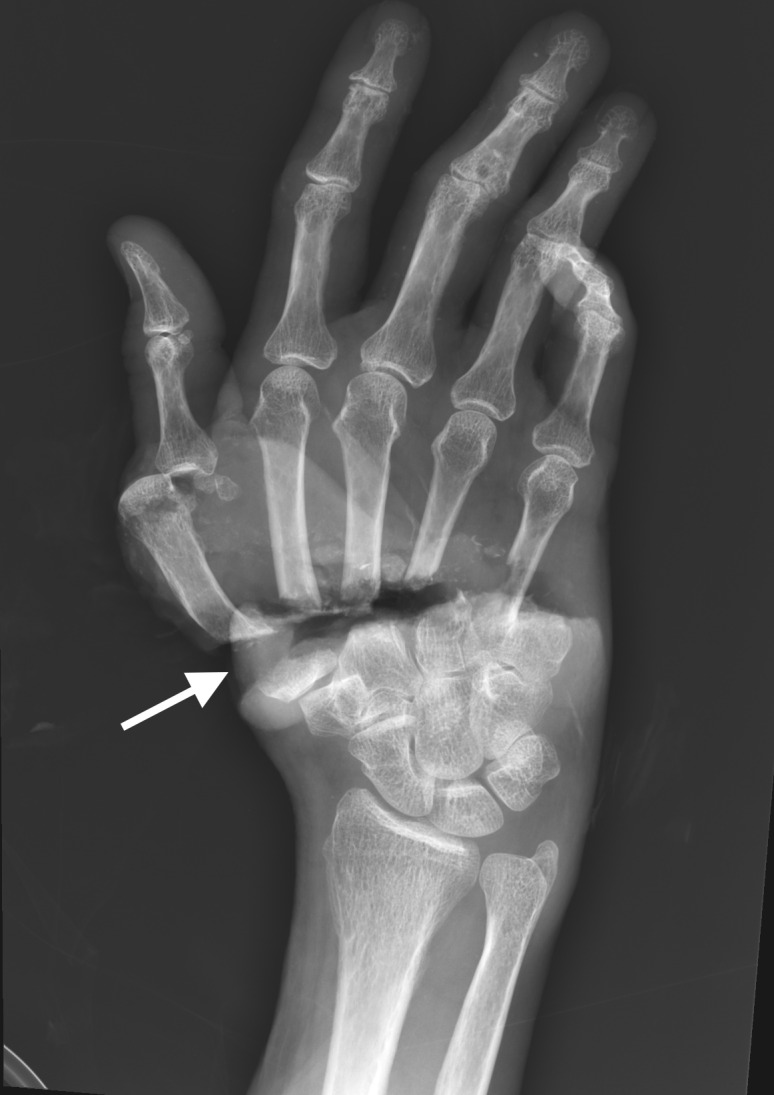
Apical 4-chamber ultrasound view demonstrating apical ballooning (arrow).
